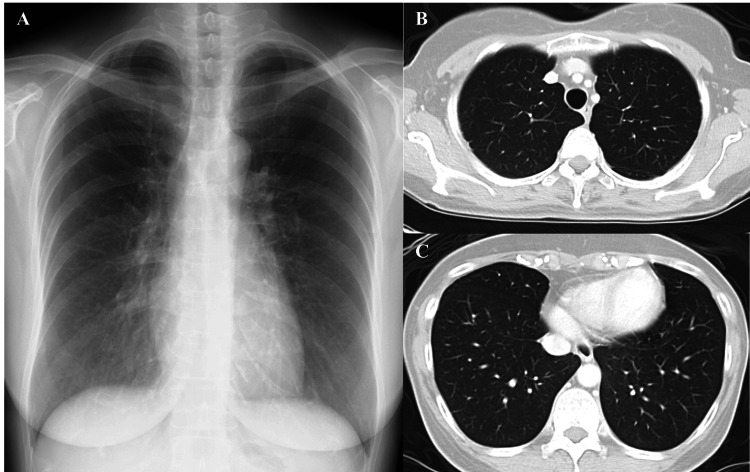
Chest imaging three months after discontinuation of mugwort steam therapy A) Chest radiograph. B, C) Chest CT scans. All previously observed abnormal opacities have resolved completely.
